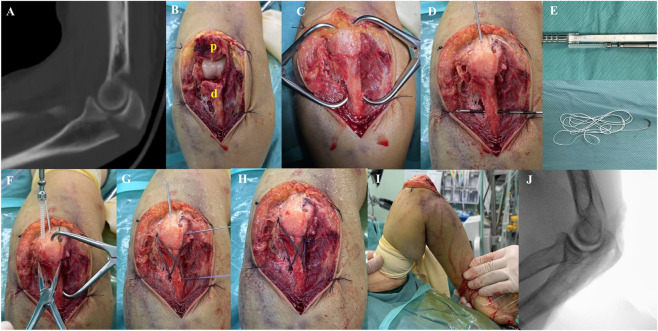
Intraoperative application of TBSASC for the treatment of (Mayo IIA). (A) Preoperative CT image; (B) Exposure of the fracture site (p: proximal ulna; (D) distal ulna); (C) Fracture reduction; (D) Establishment of transverse bone tunnel; (E) Absorbable cannulated screw and Ultrabraid high-strength suture; (F) Two 4.5 mm absorbable cannulated screws; (G) Figure-of-eight fixation with double-strand high-strength suture; (H) Post-fixation status; (I) Passive flexion and extension of the elbow joint; (J) Postoperative X-ray image.
